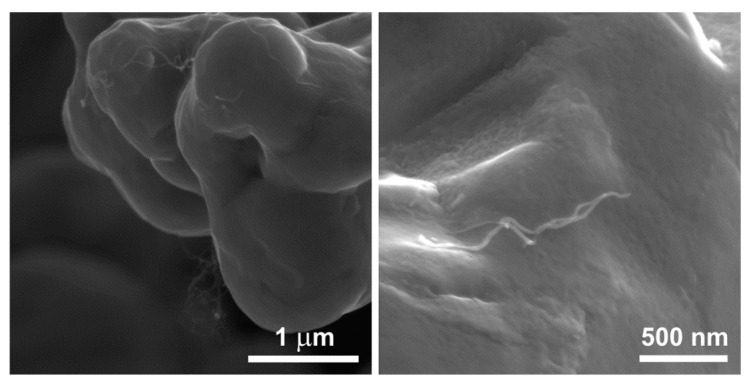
The structure of the 0.5 wt.% MWCNT/Cu powder mixed in an ultrasonic chamber and a ceramic mortar grinder.
